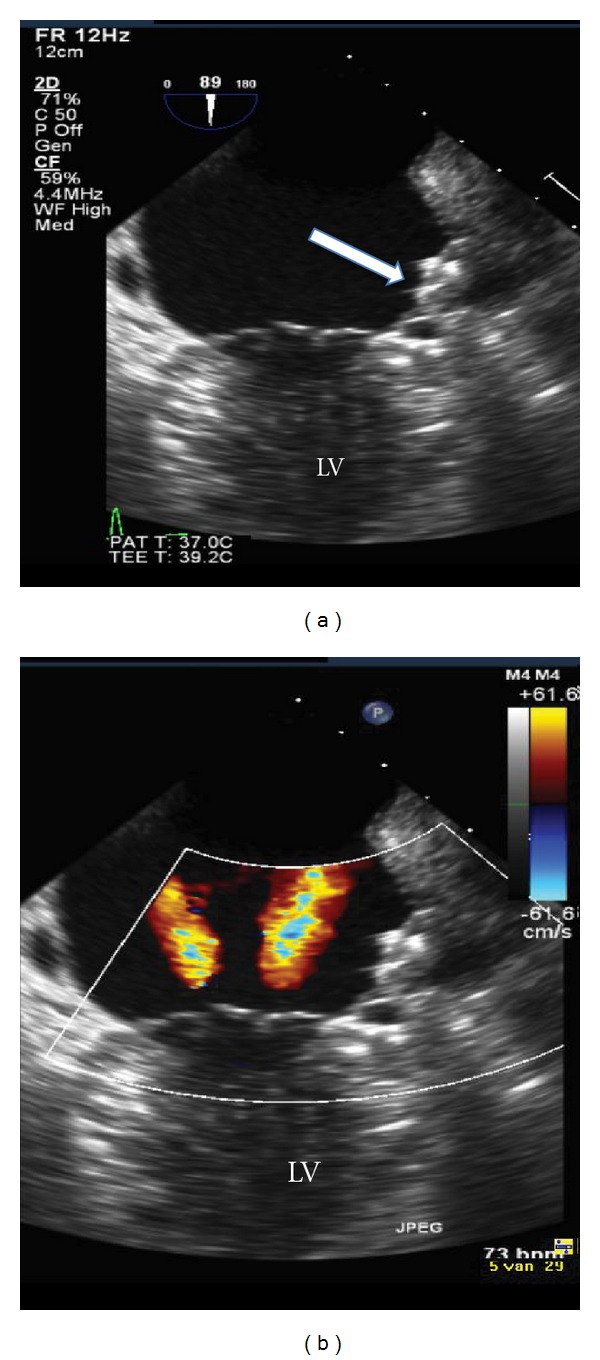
Two-dimensional transoesophageal echocardiographic imaging of the left atrium at 45-days followup. (a) Successful occlusion of the LAA without thrombus formation on the atrial surface of the device despite the limited use of anticoagulation therapy (aspirin only). (b) No residual flow around the device. LV: left ventricle.
